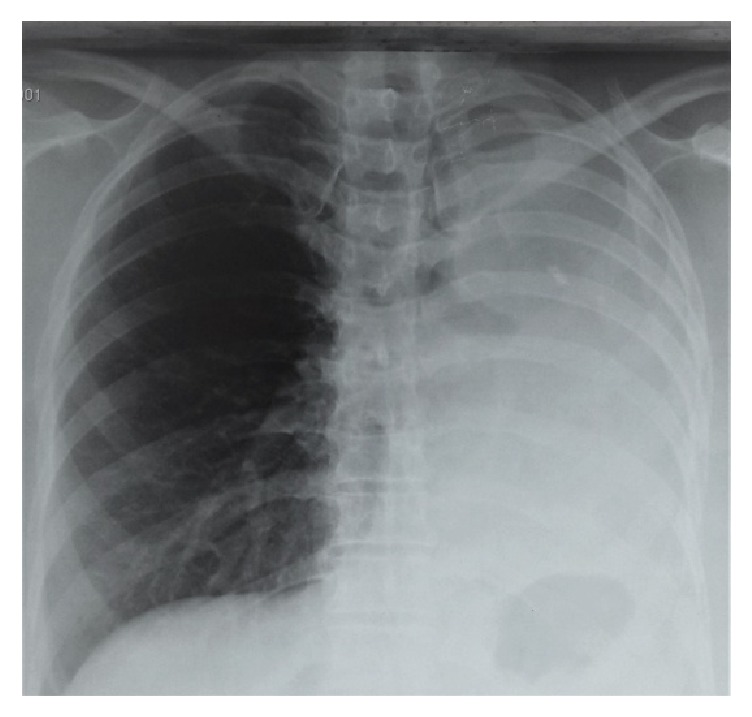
Chest X-ray showed a large opacity of the entire left hemithorax with attraction of the mediastinum element towards the opacity.
